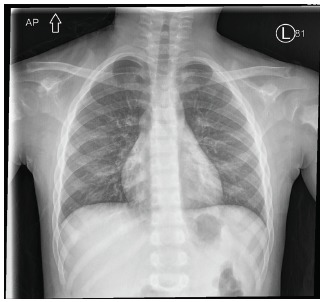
Chest radiograph of eight-year-old boy with new-onset seizure.
